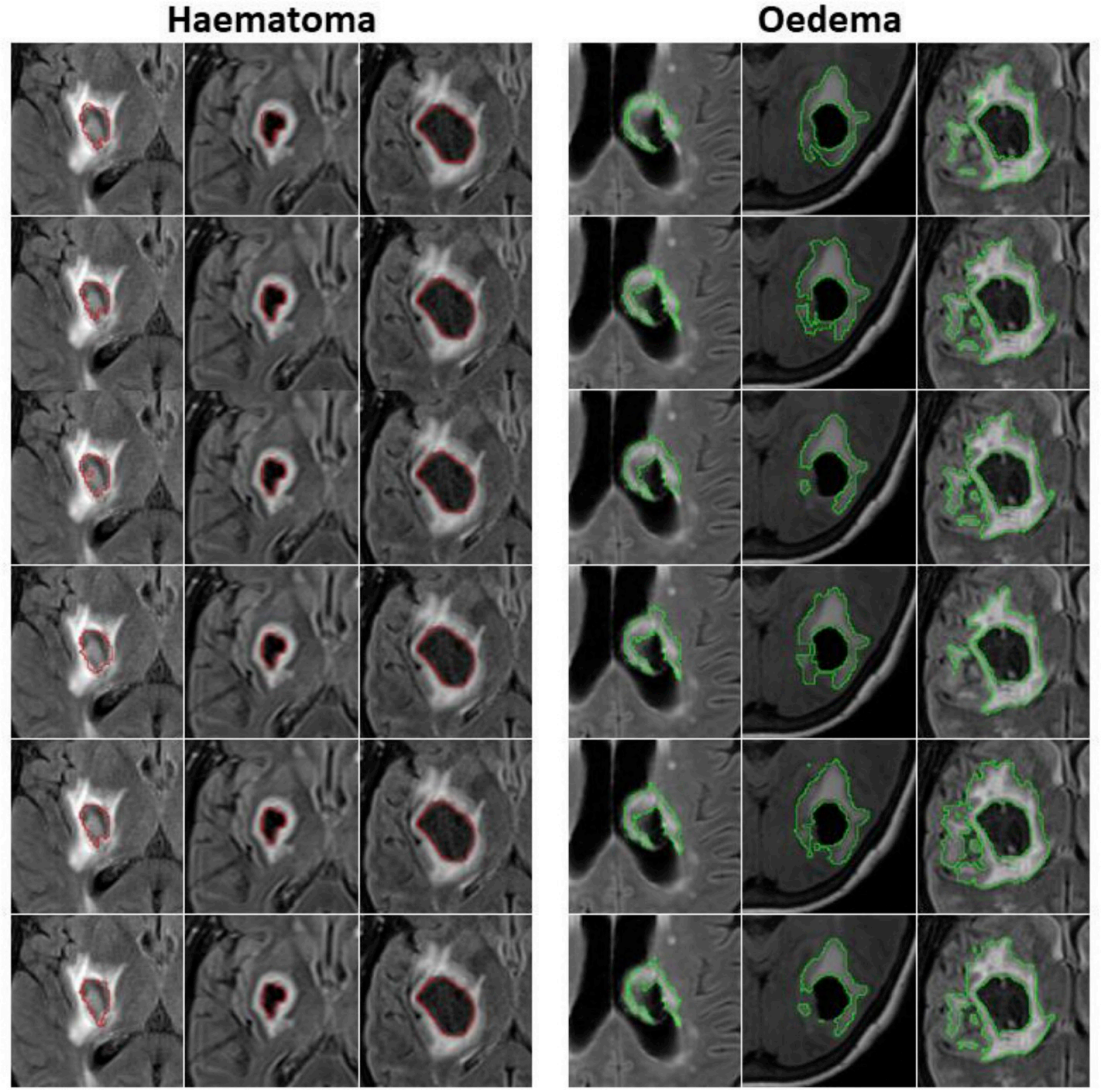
Visualisation of haematoma and perihaematoma oedema (λ=15) segmentation on Dataset A, overlaid on the FLAIR image. Worst case (left column), median case (middle column) and best case (right column), considering the average Dice score over all raters. Manual segmentations in rater order from top to bottom, with the bottom row being the proposed automated segmentation. Best viewed in colour.
